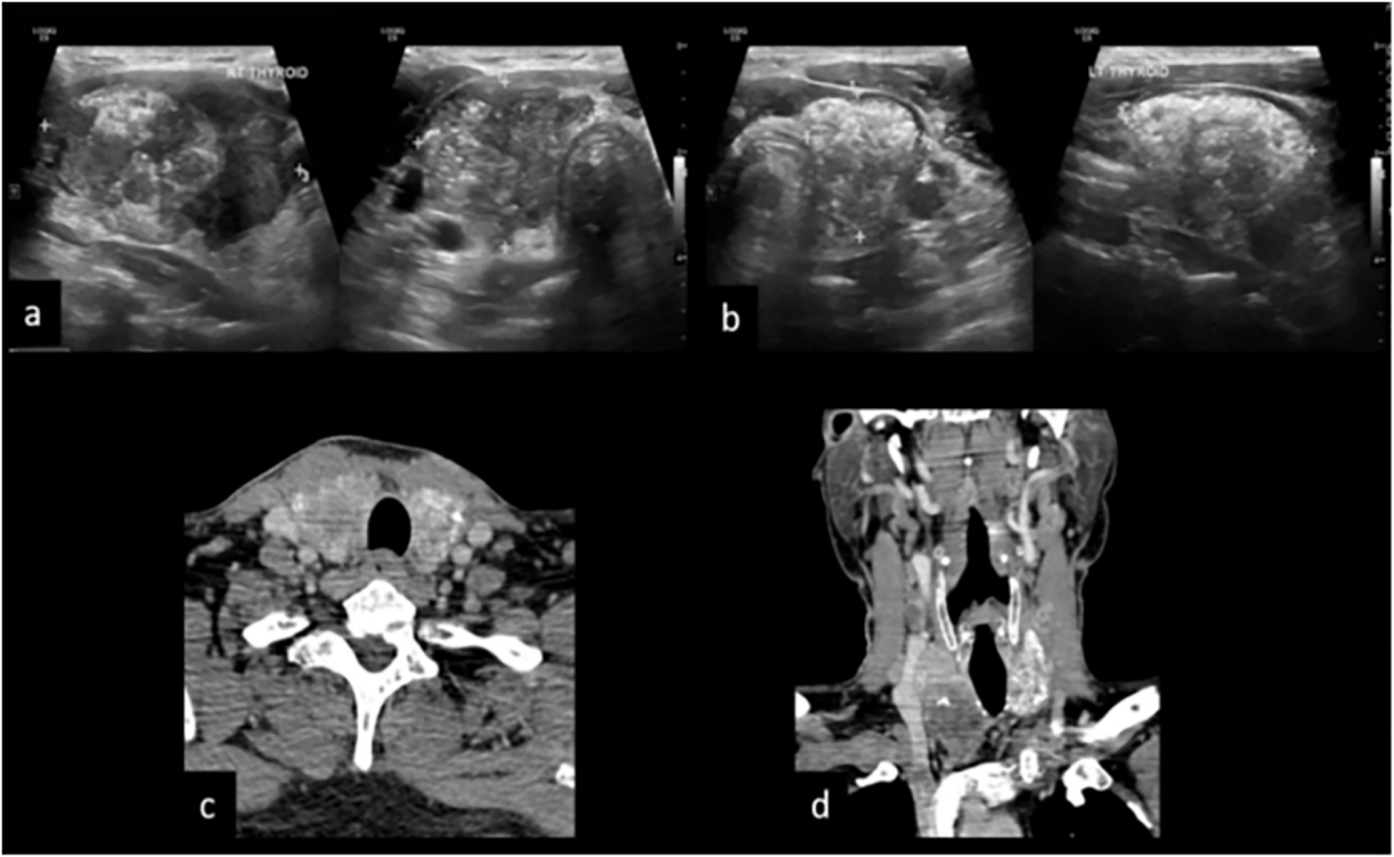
A 50-year male patient presented with anterior neck swelling since 4 months. (A, B) US reveals diffusely enlarged thyroid gland replaced completely by ill-defined solid hypoechoic mass with microcalcifications and macrocalcifications, both central and peripheral vascularity and TIRADS 5. (C) CT axial image shows heterogeneously enhancing mass replacing both the lobes of the thyroid gland with calcifications and cystic areas grade III, tracheal invasion grade I, and degree of encirclement grade IV with TE groove involvement. (D) CT coronal image shows mediastinal extension of the mass and metastatic cervical adenopathy.
